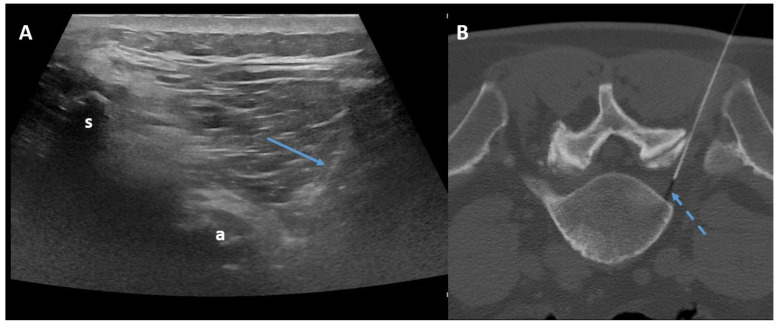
Ultrasound-guided (Panel A) and CT-guided (Panel B) lumbar paraganglionic drug injection. Blue arrow = needle visualized on ultrasound. Dotted blue arrow = ganglion root. s = spinous process, a = posterior articular complex.
